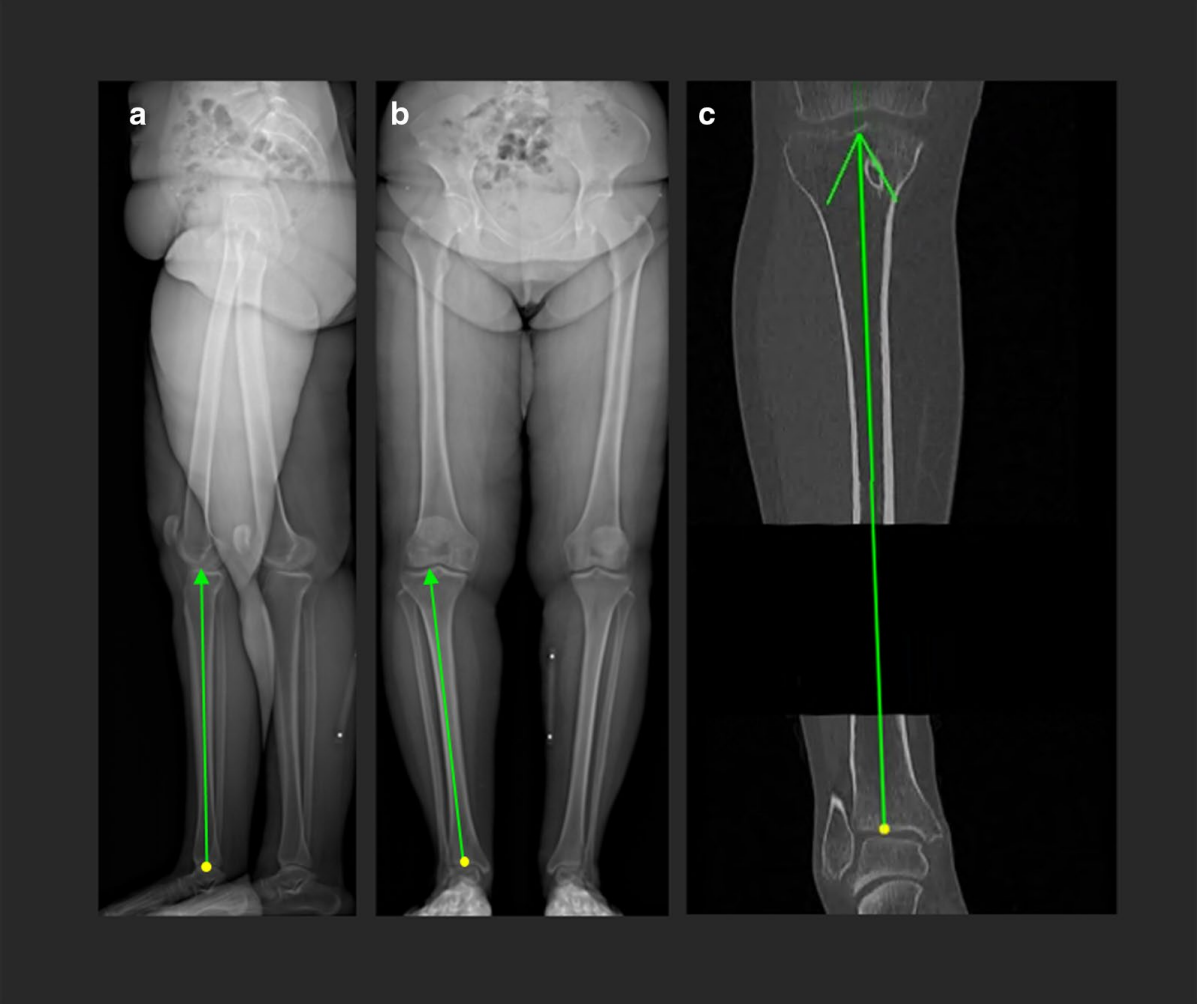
Manual annotations to initialize the registration algorithm. Three user selected annotations are used to find the initial position between EOS and DRR. In this example, an arrow is drawn from the distal to the proximal end of the tibia in the two EOS images (a, b) as well as the CT scan (c)
